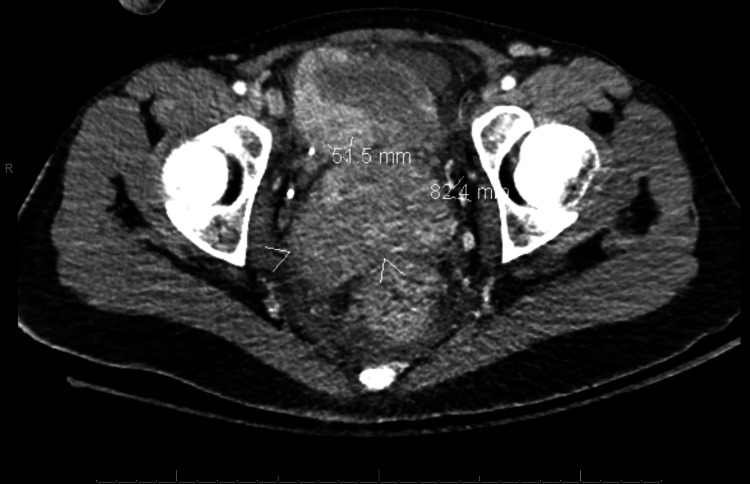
Axial CT scan displaying cervical mass White arrowheads bracket the cervical mass.
